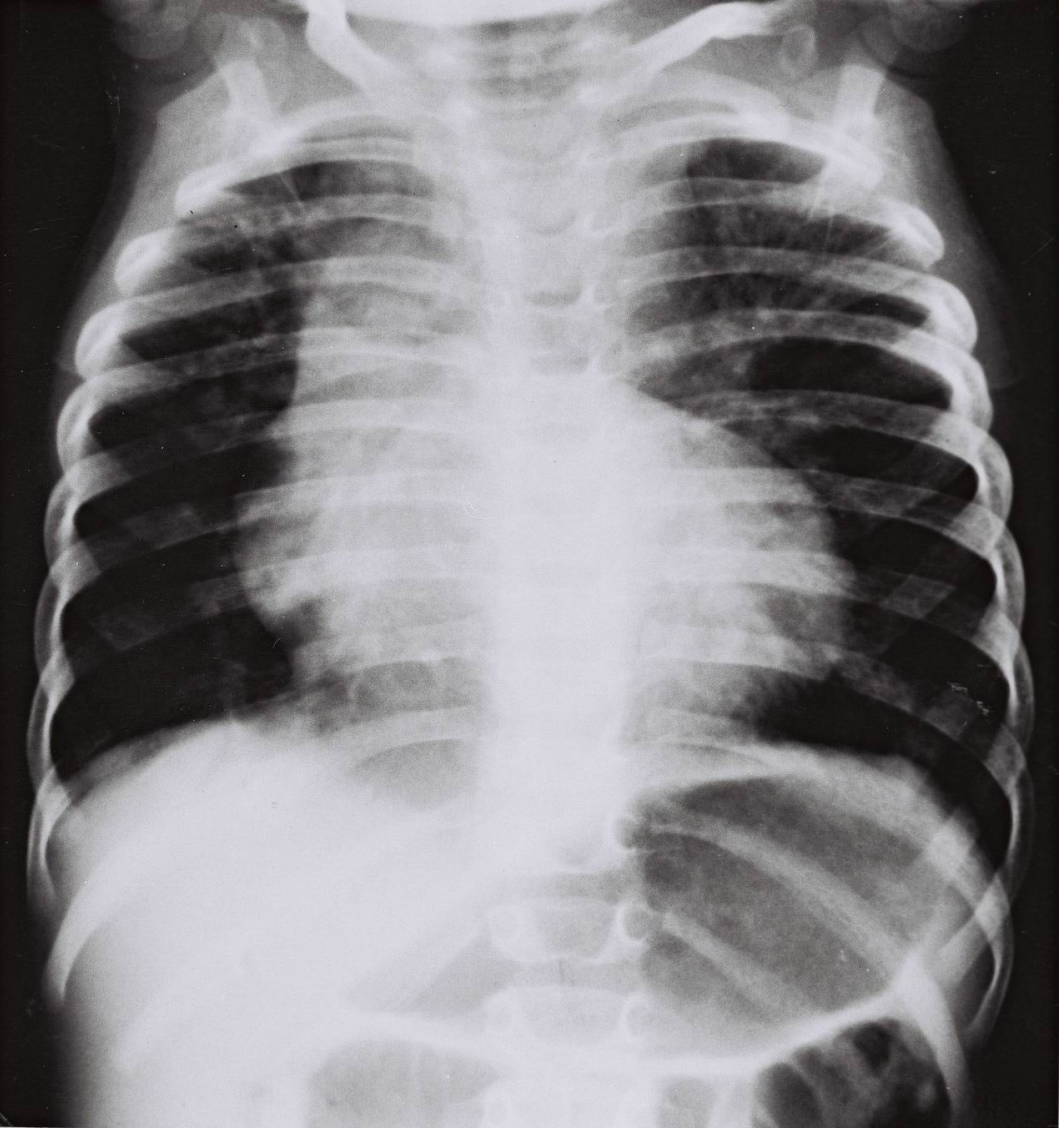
Chest radiograph of a normal girl aged 18 months, showing the difference of the shape of the thorax compared with scoliotic one (see figure 2).
